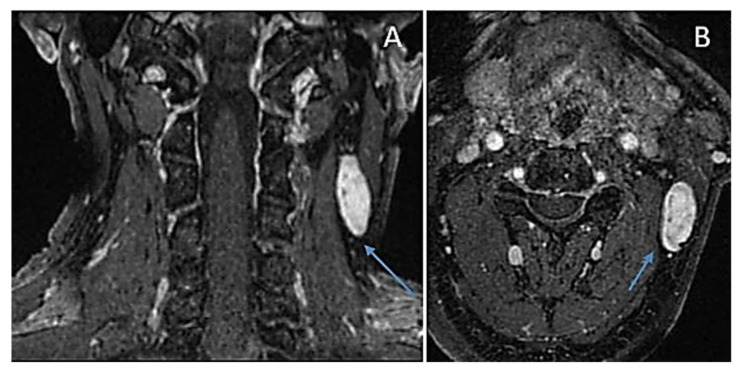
Solitary schwannoma of accessory spinal nerve. LAVA post-enhanced MRI: coronal view (A) and axial view (B) showing a non-homogenous well-defined mass (arrows) strongly enhancing along the tract of the accessory spinal nerve.
